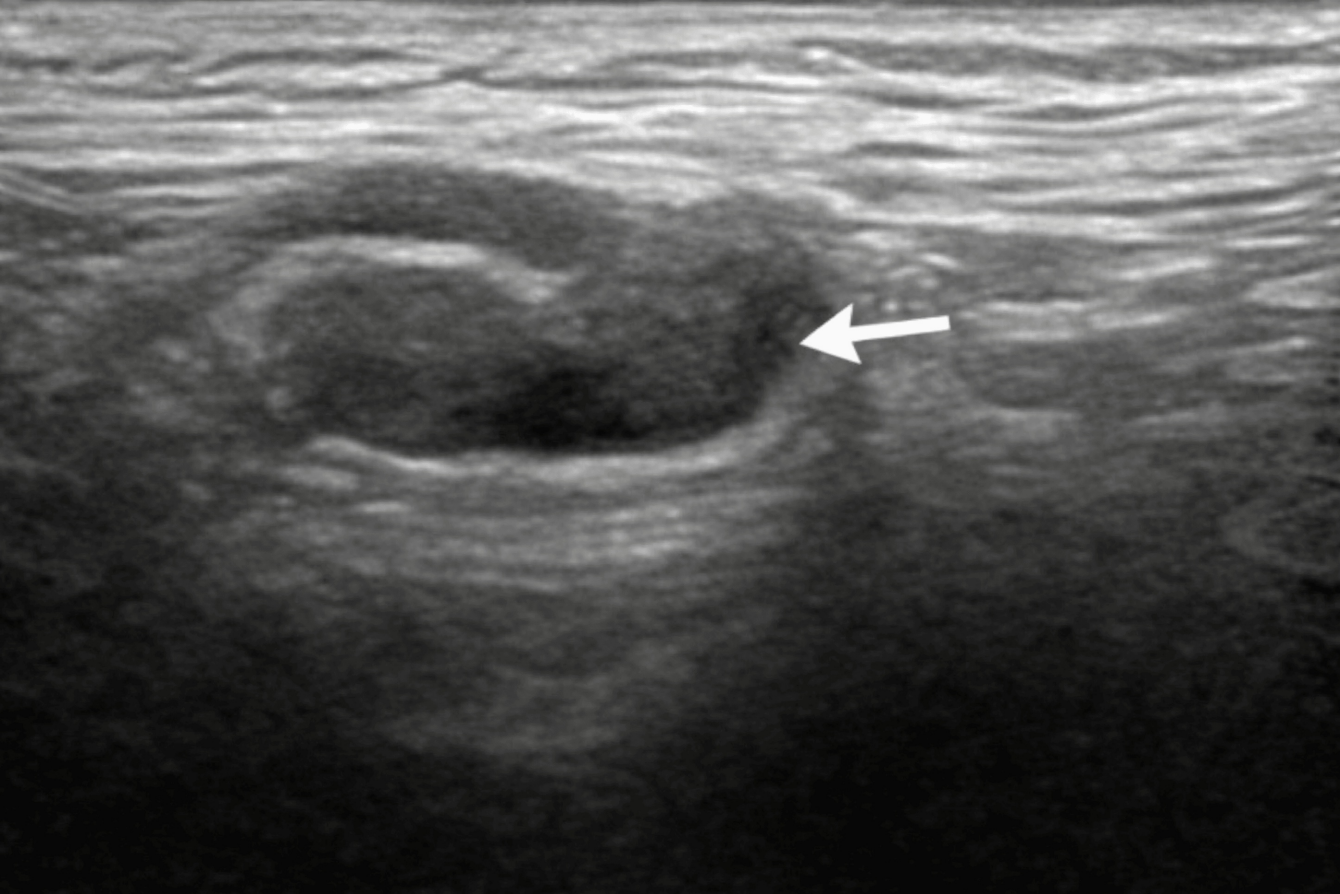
Targeted ultrasound of the left axilla demonstrating an abnormal lymph node with cortical thickening and loss of fatty hilum (arrow).
